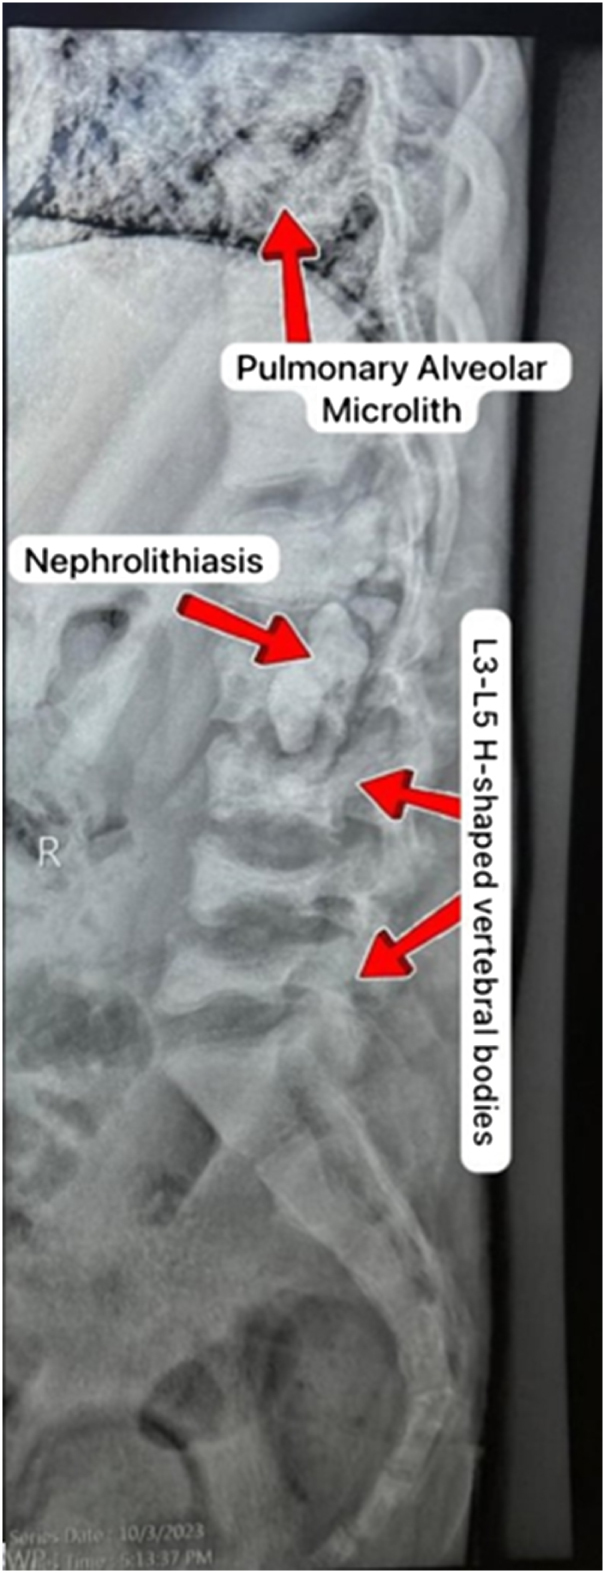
X-ray showing pulmonary alveolar microlith, nephrolithiasis, and L3–L5 H-shaped vertebral bodies.
